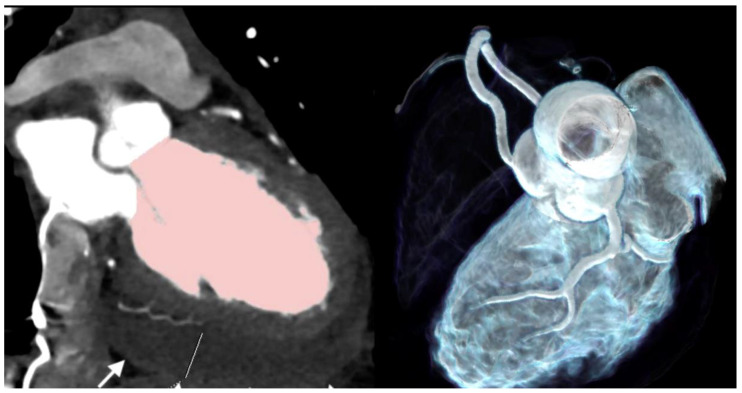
CT images (left), white arrow pointing at pericardial effusion (sagittal oblique plane), and an enlarged LV ((right), 3D VRT) with severe hypokinesis, an LV-EF of 20%, and normal coronary arteries (left).
